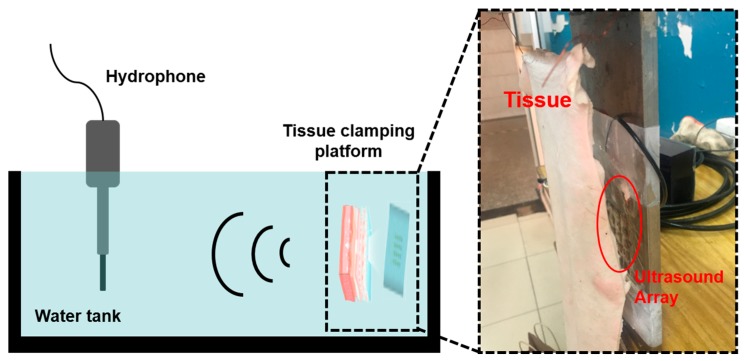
The test device of the penetration performance of ultrasound.
